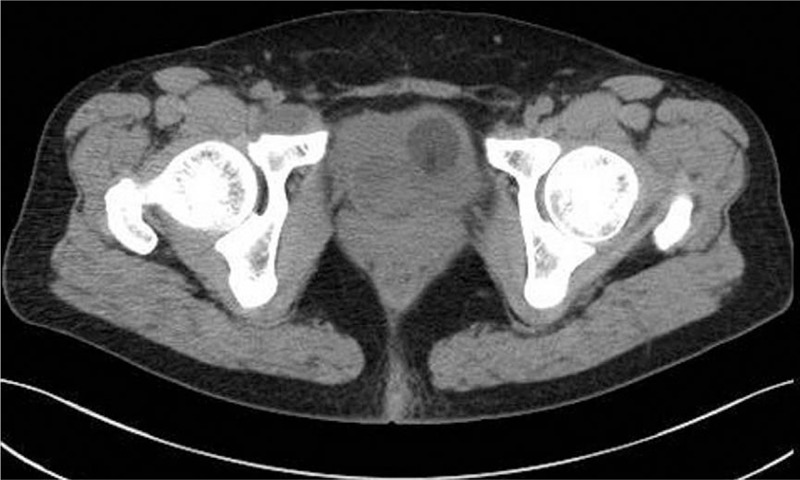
Pelvic computed tomography (CT) revealed a rounded soft-tissue mass (size of about 3.0 × 3.0 cm^2^) with a homogeneously hypodense shadow on the wall of the urinary bladder on the right side, and no abnormal pelvic lymph nodes were observed.
